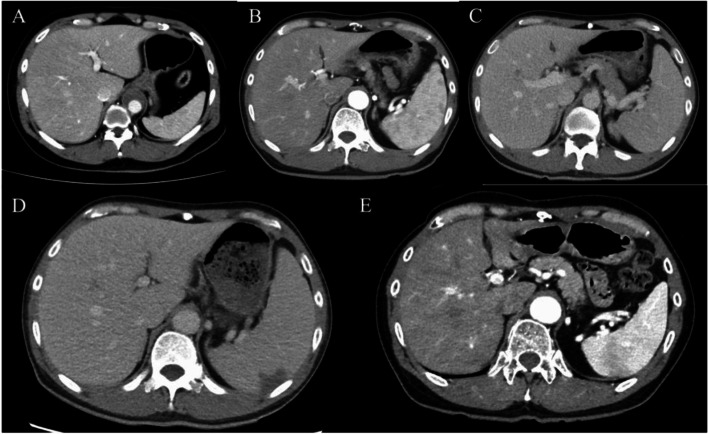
Spleen size followed up with computed tomography. Computed tomography revealed gradual enlargement of the spleen but improvement in spleen size after aortic graft replacement. A admission due to aortic dissection; B 7 months after surgery; C 13 months after surgery; D admission due to aortic graft infection 14 months after surgery; E 5 months after aortic graft replacement
